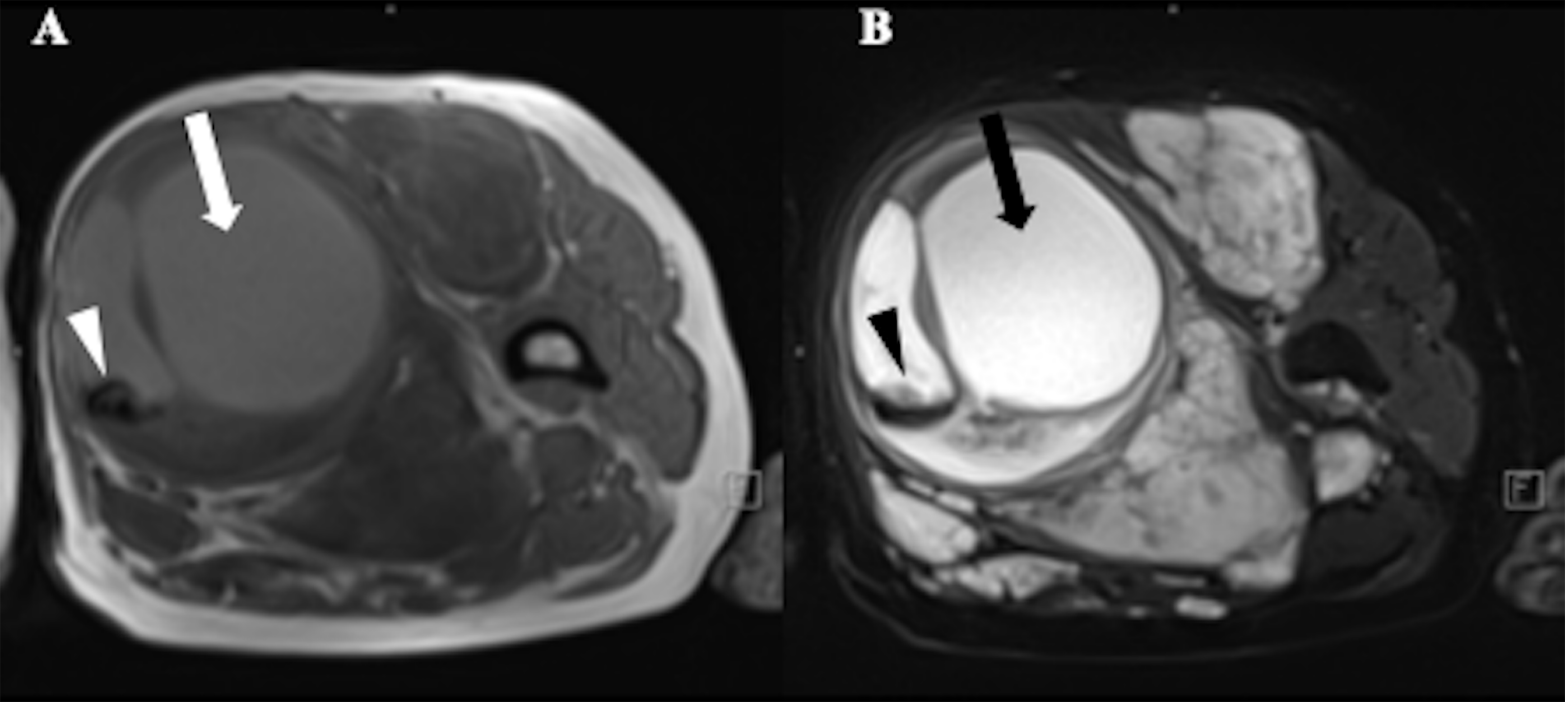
A 52-year-old female with Neurofibromatosis type 1 (NF1) who presented with a large mass in soft tissue of upper left thigh. MRI showed a heterogeneous signal with cystic change (arrows) and hemorrhage (arrowheads) in the mass, suggestive of malignant transformation. (A) T1-weighted MR image;(B) T2-weighted MR image.
